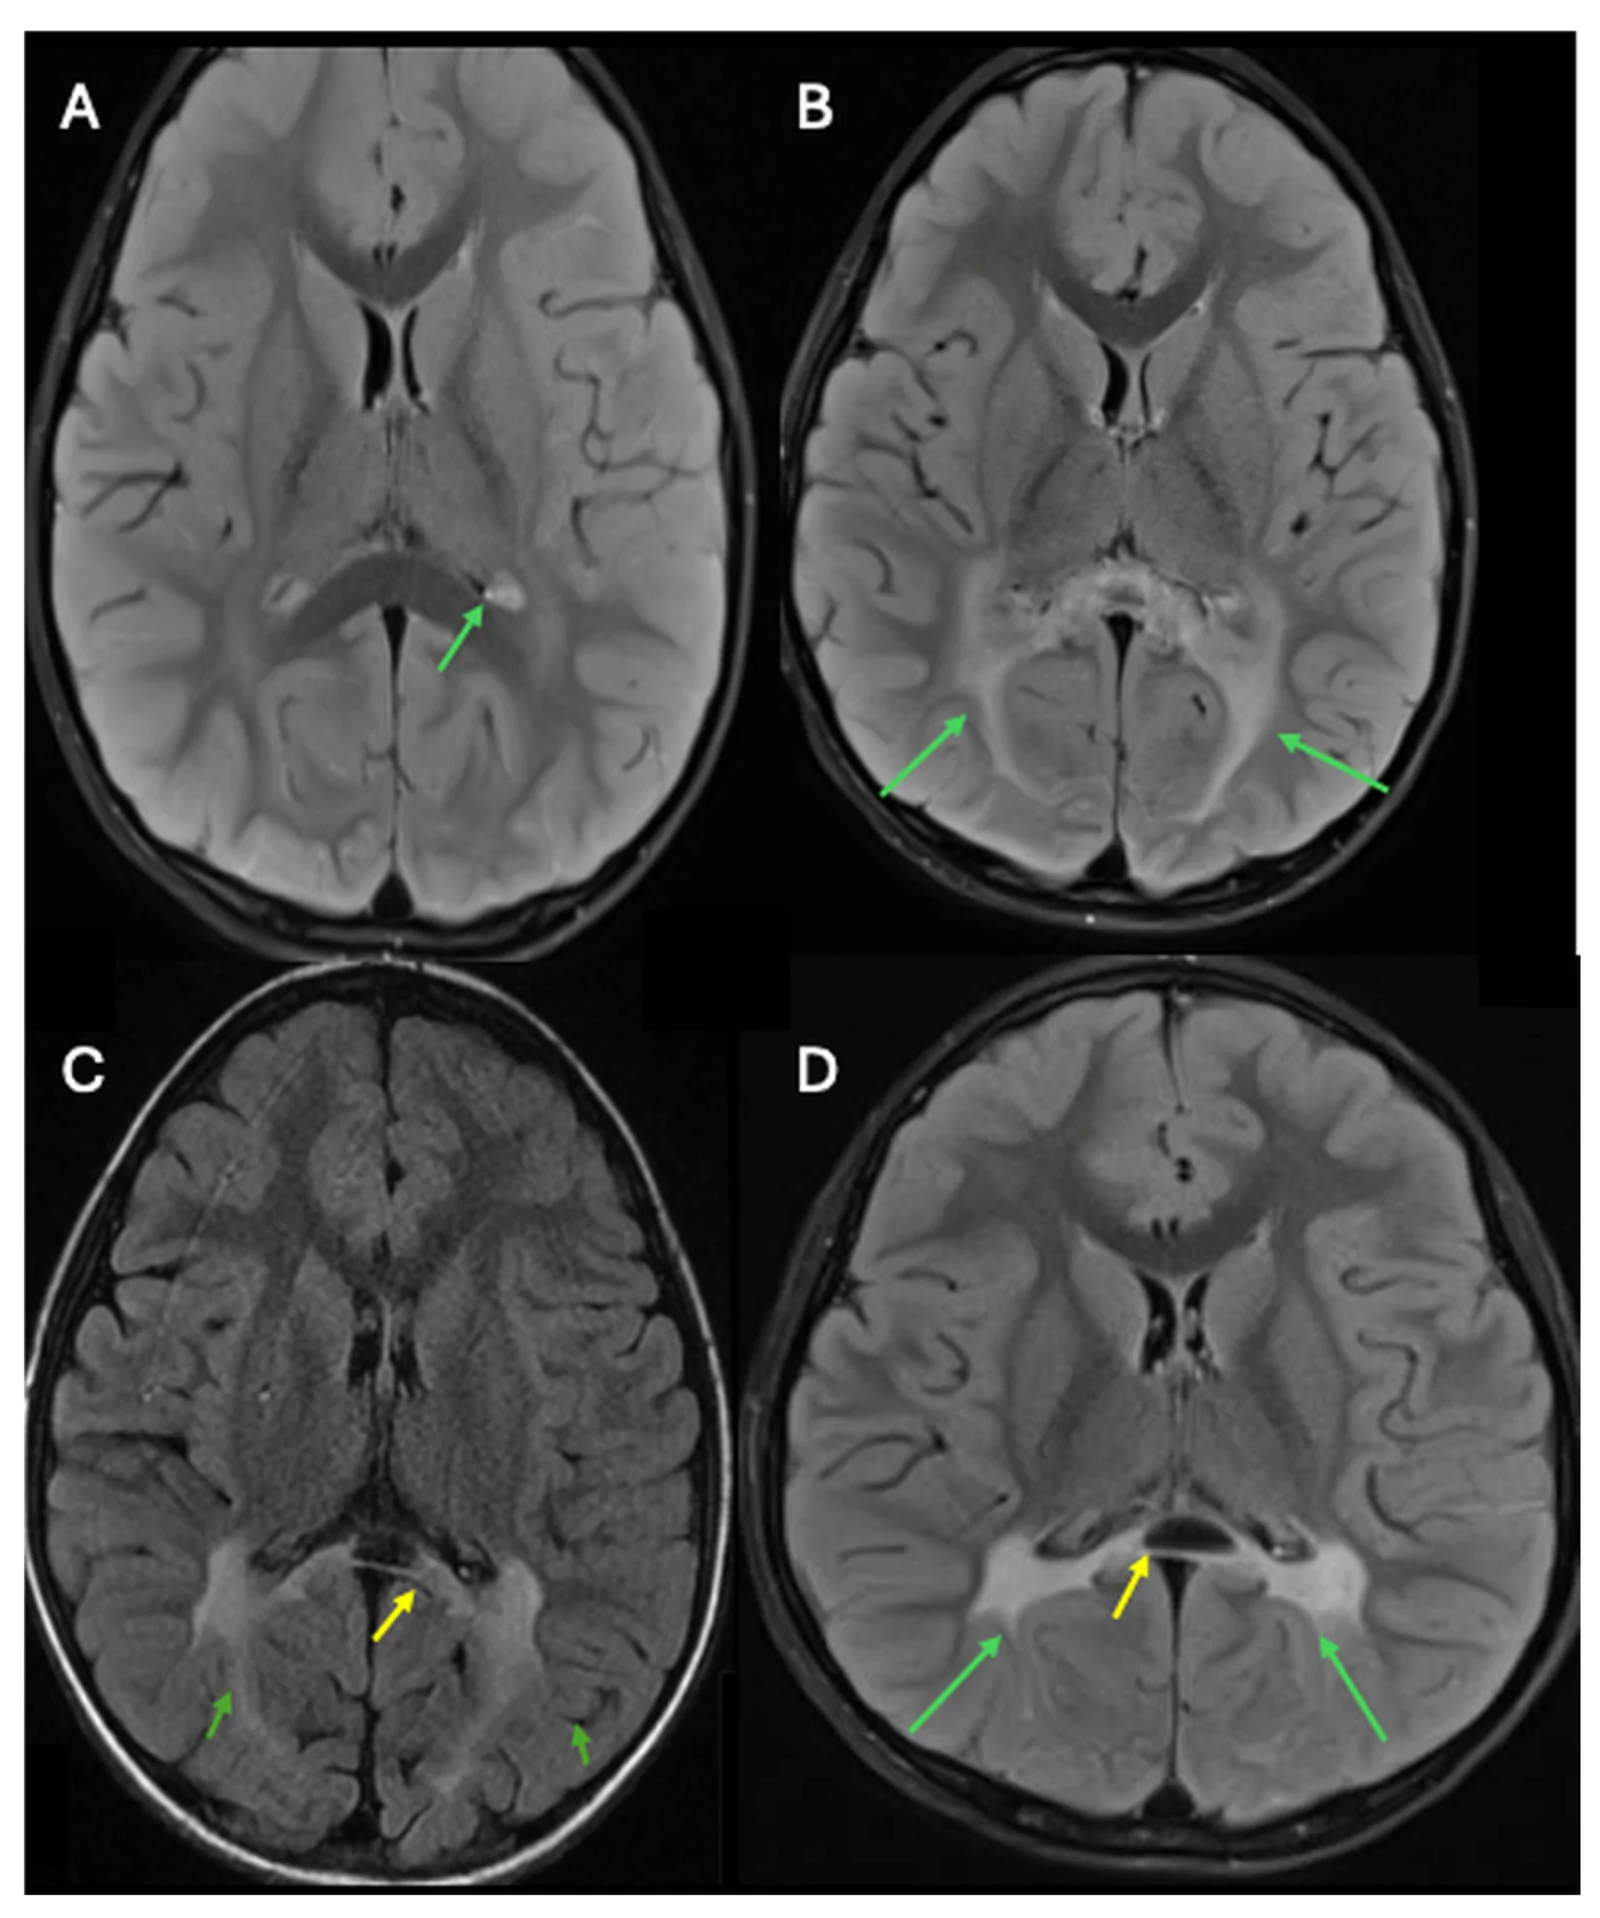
Longitudinal brain MRI demonstrating the evolution of white matter abnormalities in a patient with Krabbe disease. Axial FLAIR imaging obtained at two-and-a-half years of age demonstrates mild, symmetric bilateral periatrial white matter T2/FLAIR hyperintensity (green arrows), which was of uncertain significance and may represent either a normal variant or early manifestation of Krabbe disease (A). Follow-up axial FLAIR imaging obtained around three-and-a-half years of age demonstrates progression of T2/FLAIR hyperintensity involving the posterior periatrial white matter and forceps major (green arrows) (B). Axial T2/FLAIR imaging at 6 years of age, approximately two years following the second transplant, demonstrates stable moderate T2 hyperintense signal within the posterior centrum semiovale and corona radiata (green arrows), with interval development of cystic gliosis involving the splenium of the corpus callosum (yellow arrow) (C). Most recent axial T2/FLAIR imaging obtained at 9 years of age demonstrates stable periatrial T2/FLAIR hyperintensity involving the splenium of the corpus callosum (green arrows) and persistent cystic gliosis within the splenium (yellow arrow) (D).
